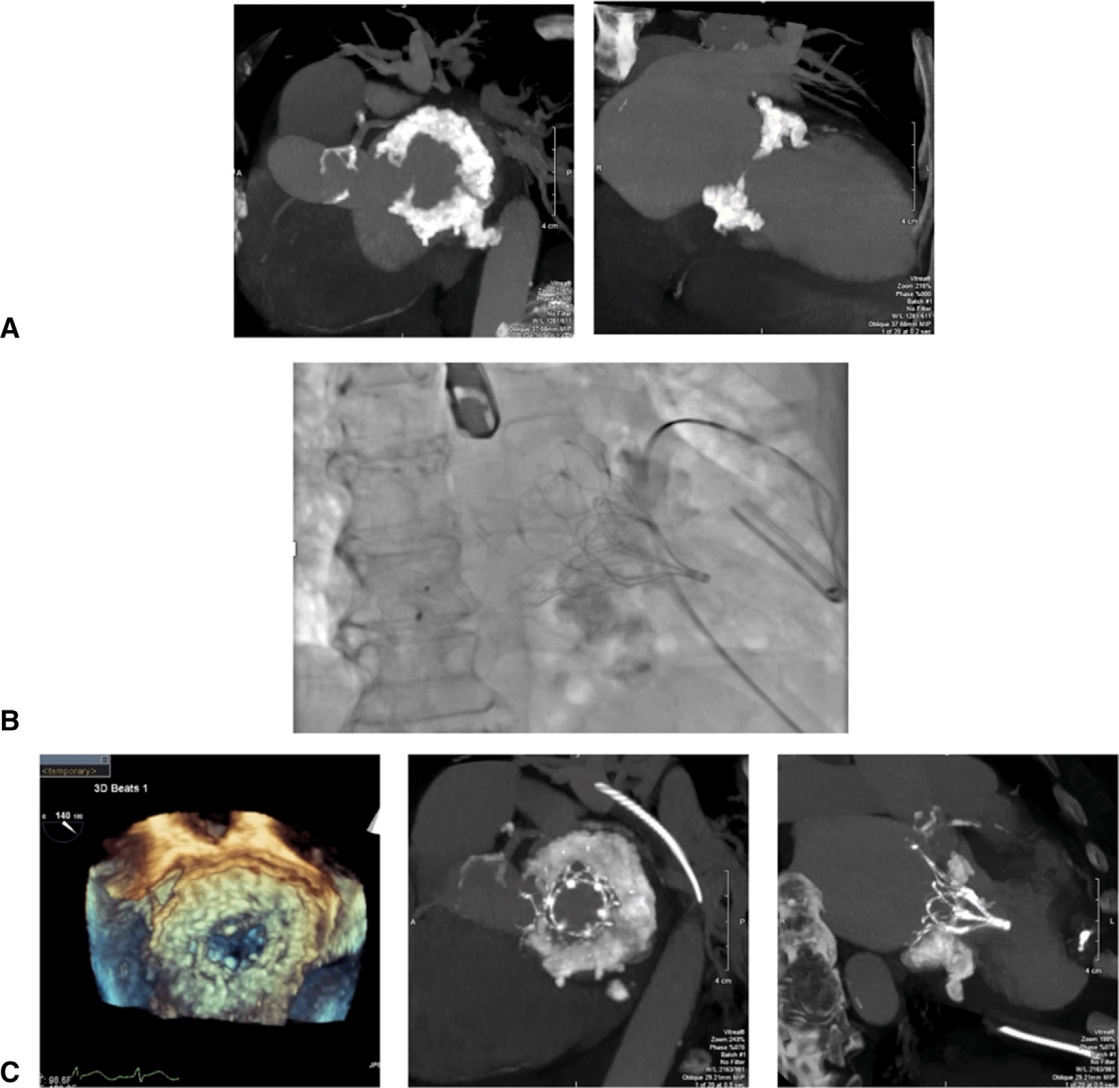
Tendyne MVR A, Preoperative CT demonstrating severe MAC. B, Intraoperative fluoroscopy demonstrating placement of Tendyne valve. C, Postoperative 3D echocardiogram and CT demonstrating placement of Tendyne valve.
